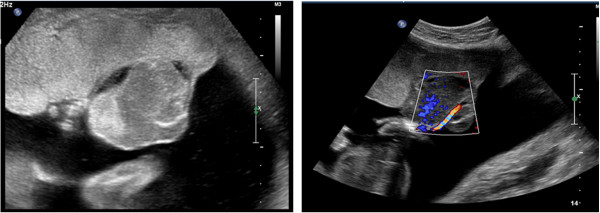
Ultrasound image of the chorioangioma before intervention.
